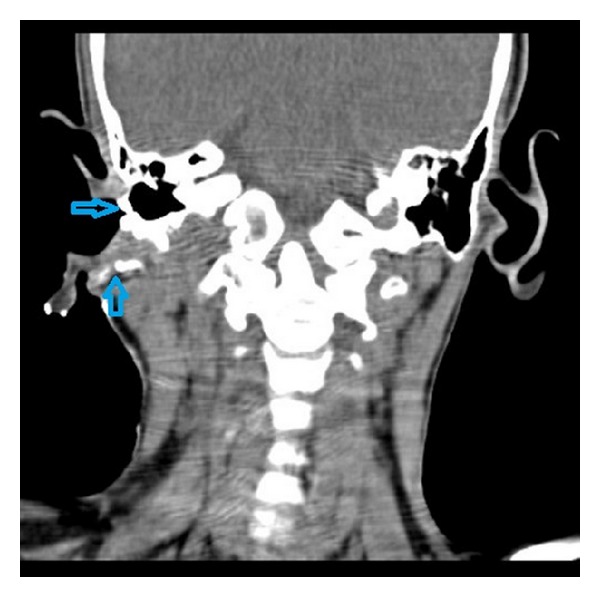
CT fistulogram with coronal reconstruction (posterior to Figure 2) showing the bifurcation of the fistula tract; one opening into the external auditory canal (shown by right facing arrow) and other going to retroauricular region (shown by upward arrow).
